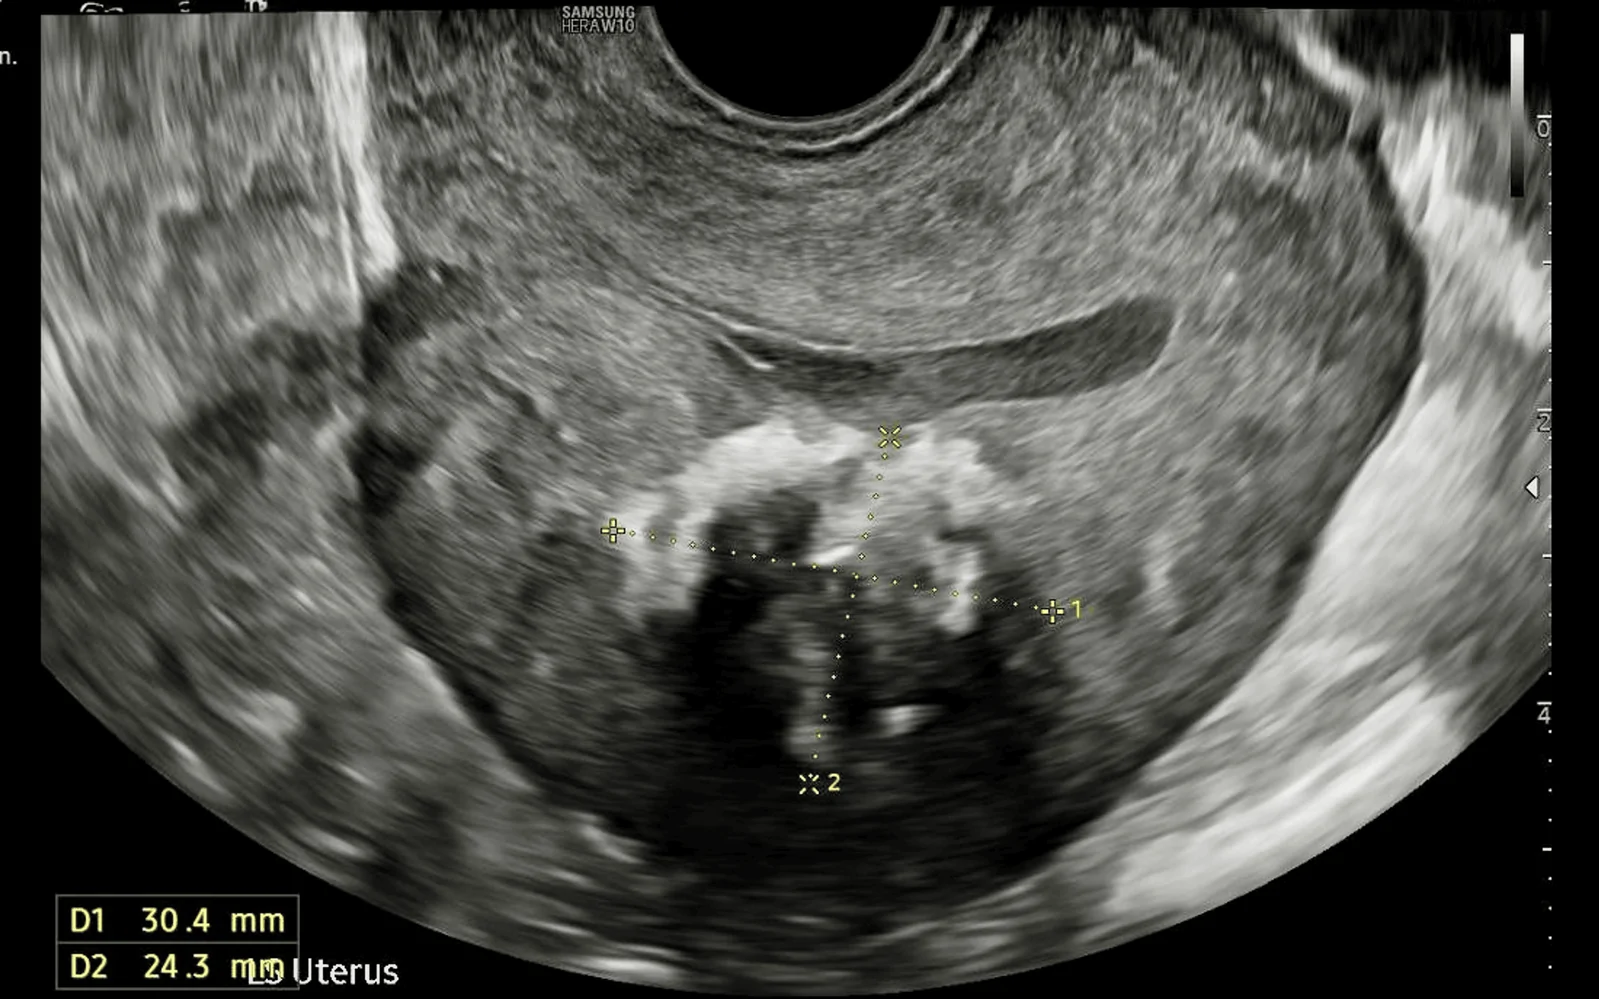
Follow-up ultrasound after spontaneous fibroid expulsion showing marked interval reduction. Follow-up ultrasound performed one month after spontaneous vaginal expulsion of the fibroid, demonstrating marked interval reduction in size, with a residual anterior fibroid adjacent to the endometrial cavity measuring approximately 30 × 24 × 34 mm.
